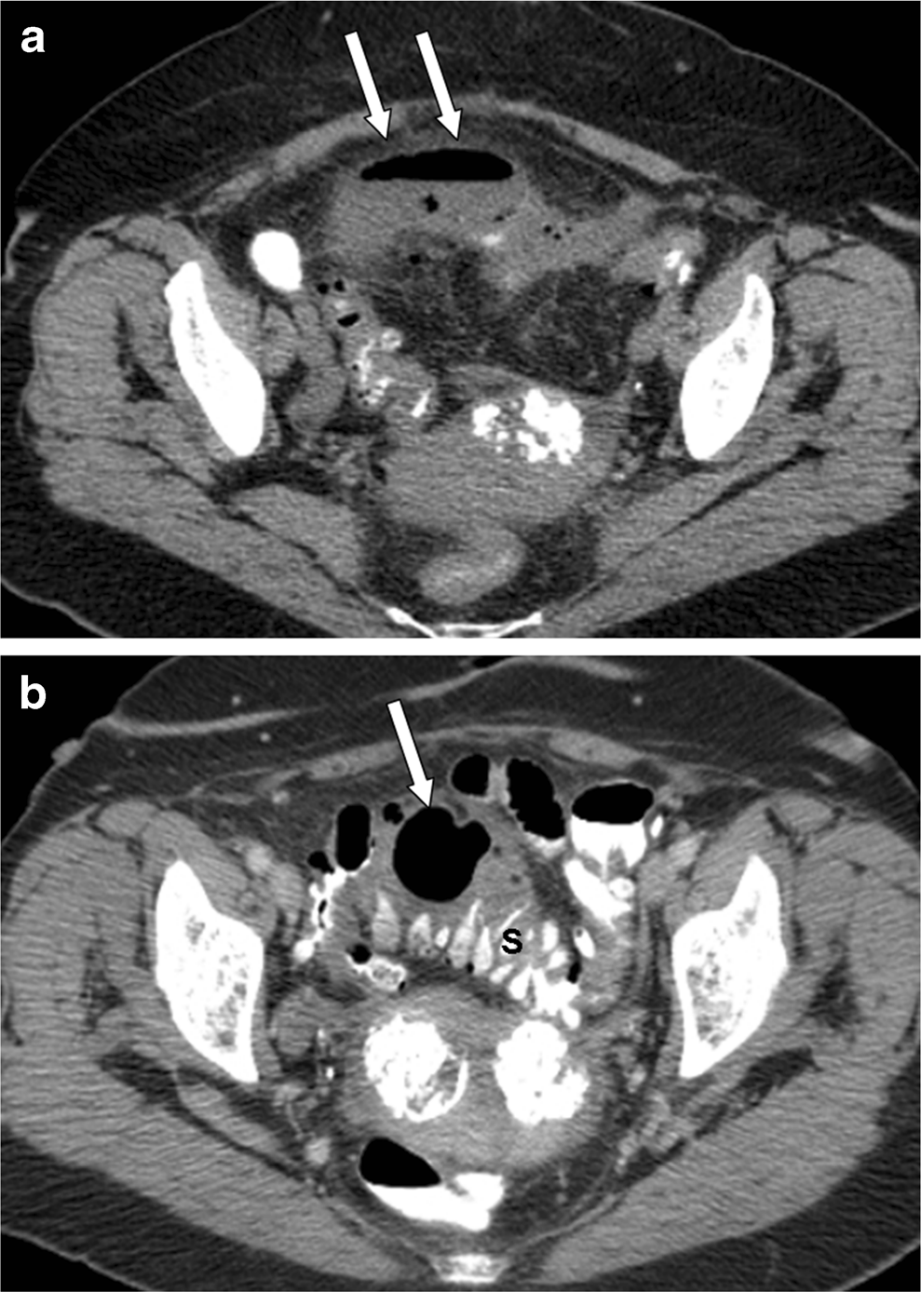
An 83-year-old woman with type II (inflammatory type) giant sigmoid diverticulum, which developed after an episode of acute diverticulitis. Axial (a) contrast-enhanced CT image of the abdomen shows a peridiverticular abscess (arrows), which was percutaneously drained. Axial (b) contrast-enhanced CT image obtained 5 months later (follow-up CT examination) shows a gas-filled structure adjacent to the sigmoid colon (arrow), without air–fluid level or pericolic inflammatory changes, consistent with type II (inflammatory type) GCD
